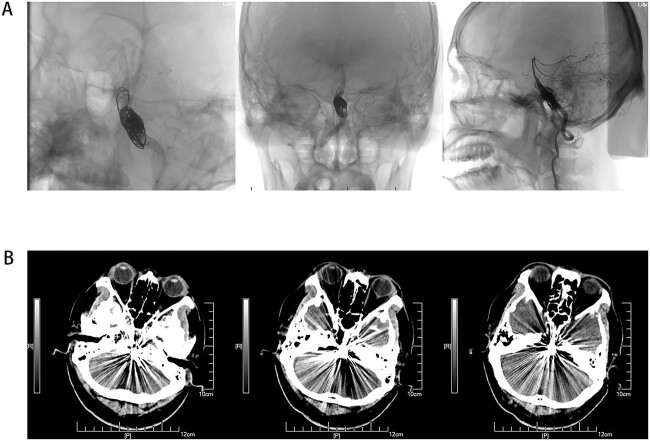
(A) Coil and stent implantation for VBD, and show peripheral blood supply after operation. (B) Non-contrast axial CT scan of the brain showing VBD after surgery.
